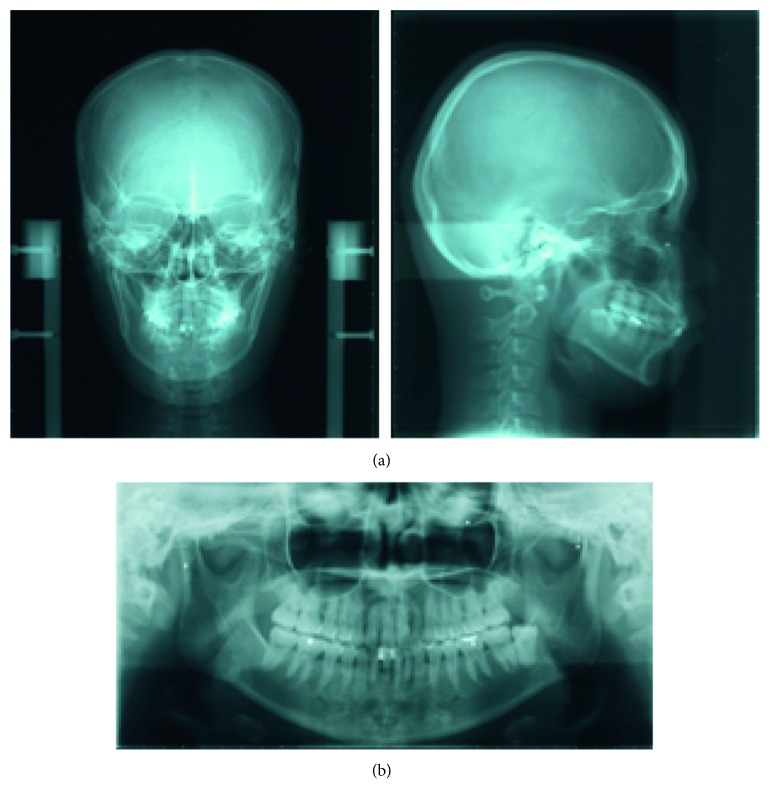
Pre-orthodontic treatment cephalograms (a) and panoramic radiograph (b) are shown.
